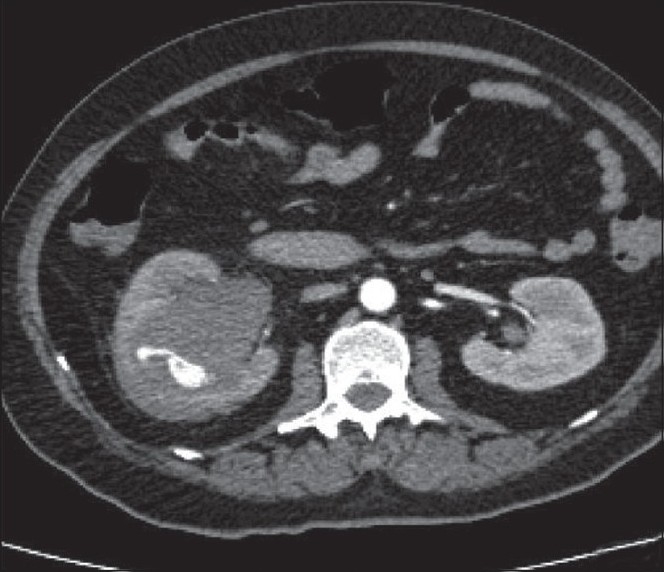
CT angiogram showing ruptured artery supplying the interpole
